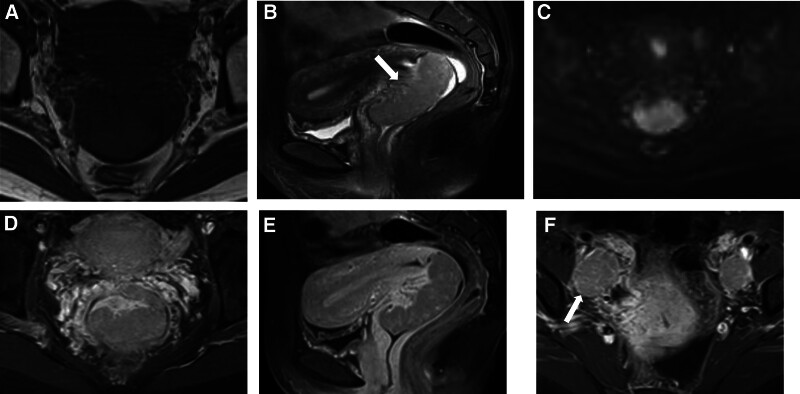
MRI image showing clear cell adenocarcinoma of the uterine cervix. Axial T1 weighted MR image (A) shows a lesion in the uterine cervix, with a slightly hyperintense signal. Sagittal T2 fat suppression image (B) shows hyperintense signal intensity and uniform cystic change of tumor (white arrow). Diffusion-weighted magnetic resonance imaging (C) shows limited diffusion in the tumor. Axial (D) and sagittal enhanced images (E) show significant tumor enhancement. Axial-enhanced image (F) shows enlarged lymph nodes around the bilateral iliac vessels (white arrow).
